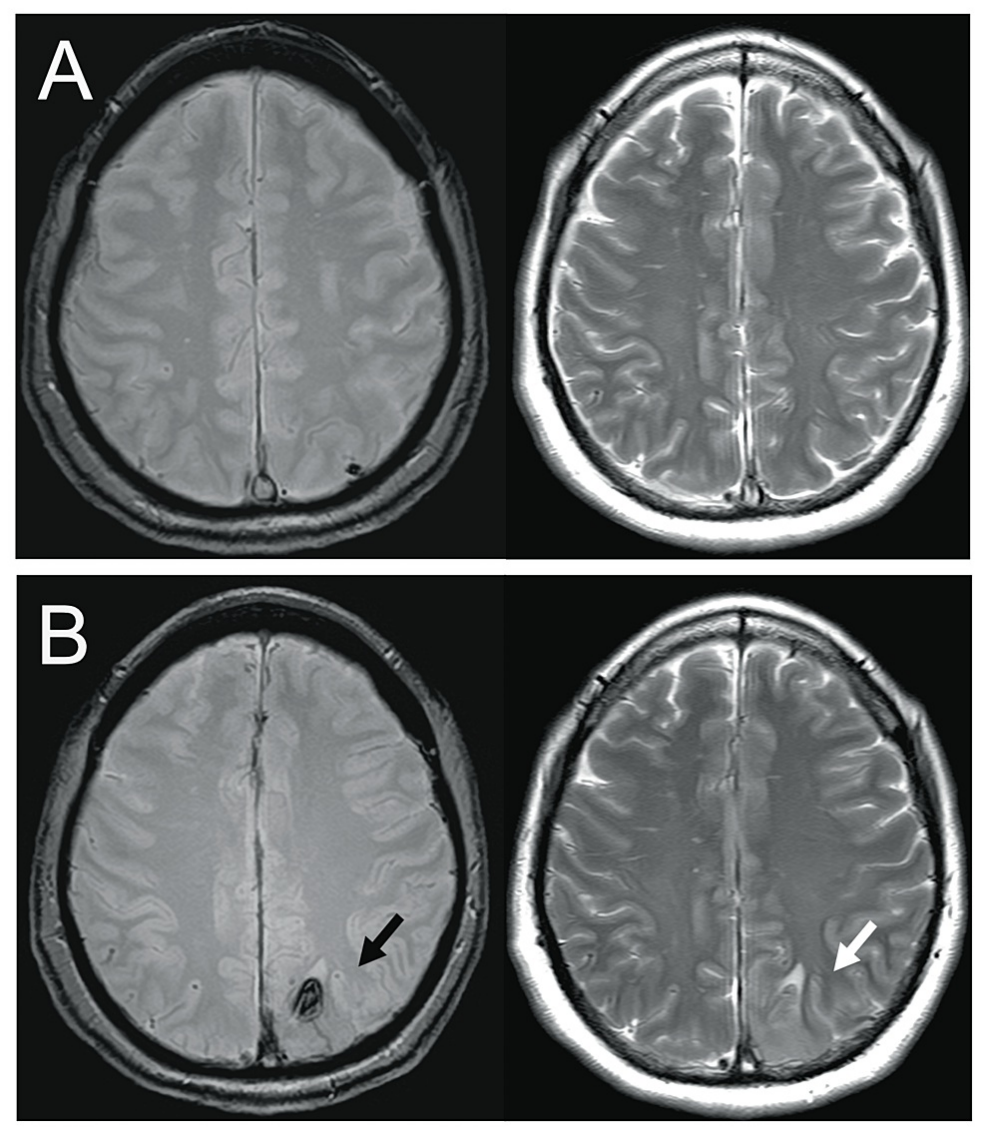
Time series analysis of exacerbation of CVST after heparinization Axial T2* (left) and T2 (right) weighted images on the seventh (A) and 12th day (B) after heparinization for CVST are shown. MRI remained unchanged from the admission assessment without clear evidence of venous infarction (A). However, on the 12th day when HIT developed, MRI revealed subcortical hemorrhage (black arrow) and cerebral edema (white arrow) in the left parietal lobe, suggesting acute exacerbation of CVST (B). CVST - cerebral venous sinus thrombosis, HIT - heparin-induced thrombocytopenia
